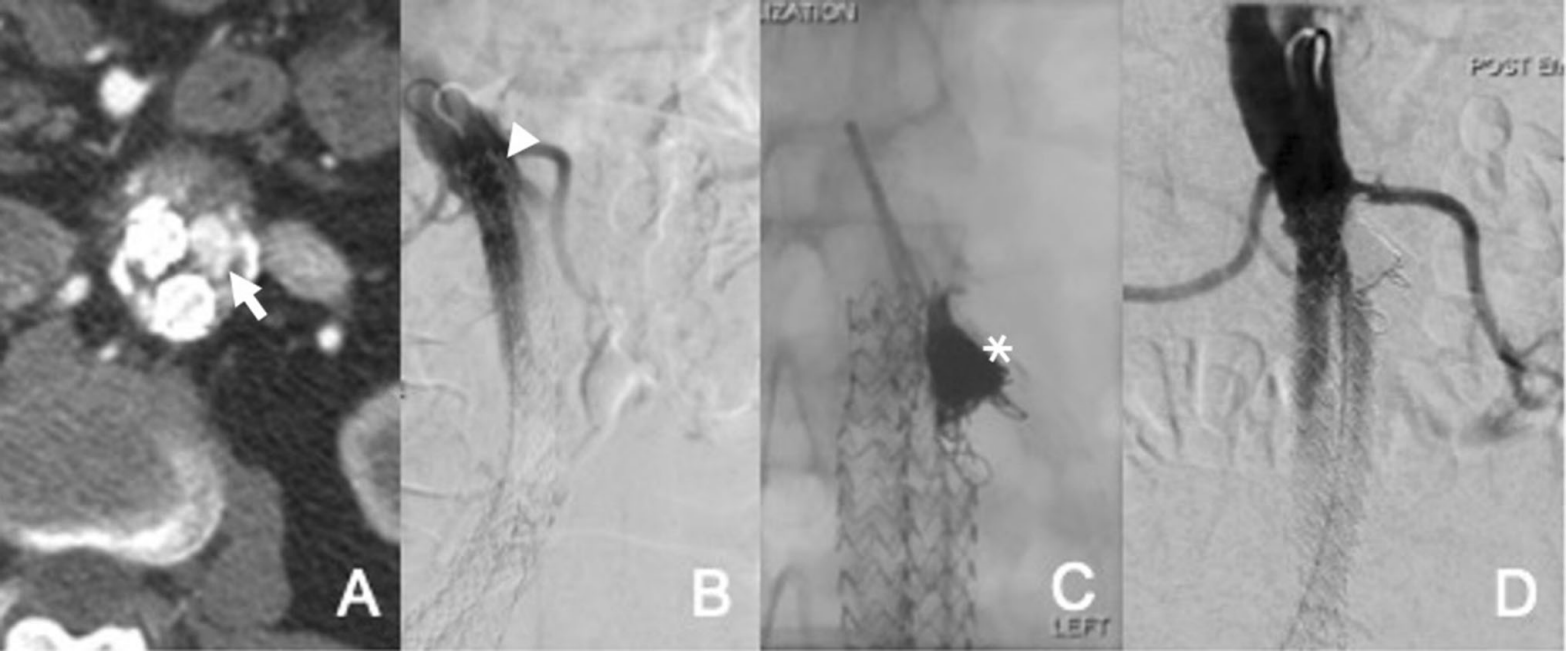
EL1a embolisation. A Axial CT angiogram shows proximal EL1 in patient with a Nellix endograft (arrow). B Aortic angiograms confirm EL1a (arrow head). C Embolisation of the endoleak cavity with coils and Onyx via a microcatheter following catheterisation of the endoleak cavity with reverse shaped catheter (asterix). D Final angiogram shows successful endoleak embolisation
